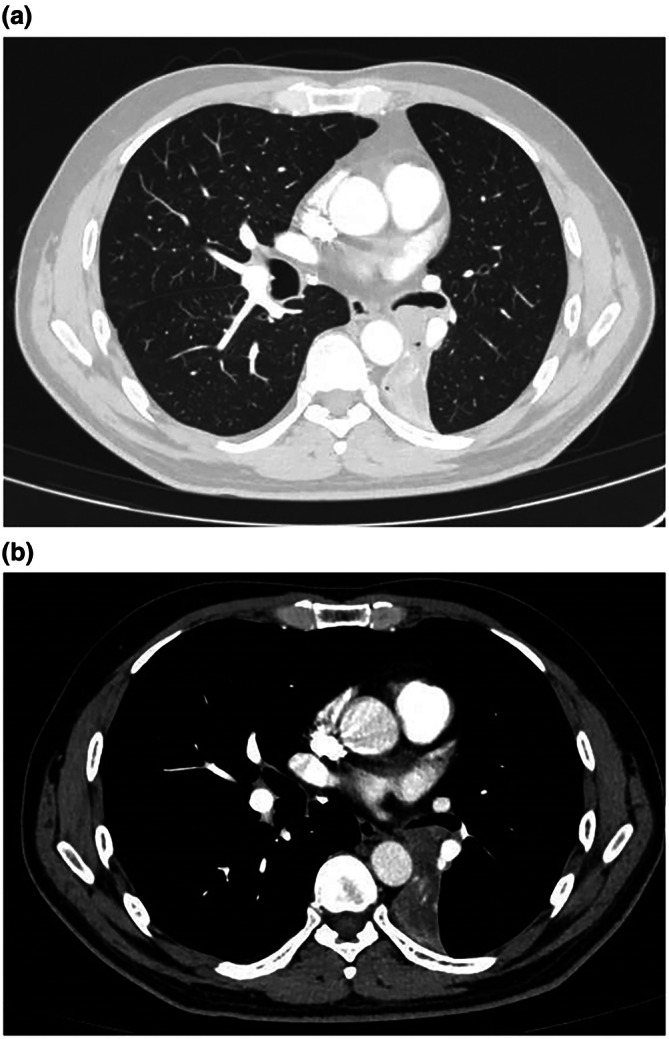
Chest‐enhanced CT scan revealing a 1.9 × 1.3 cm nodular lesion causing consolidation and atelectasis of the left lower lobe. (a) Lung window. (b) Mediastinal window.
